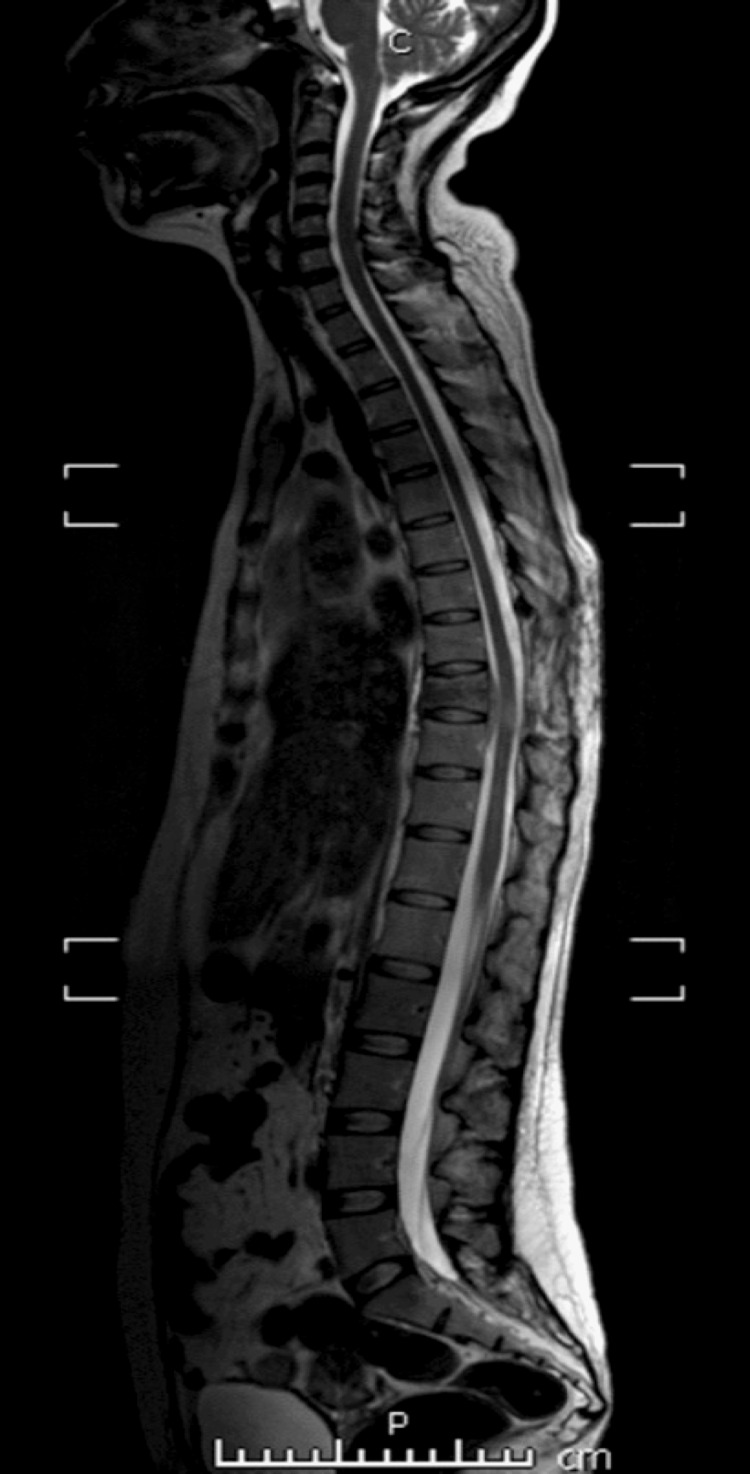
Magnetic resonance imaging of the spine after six cycles of chemotherapy with the DA-EPOCH-R regimen DA-EPOCH-R: dose-adjusted etoposide, prednisone, vincristine, cyclophosphamide, doxorubicin, and rituximab
